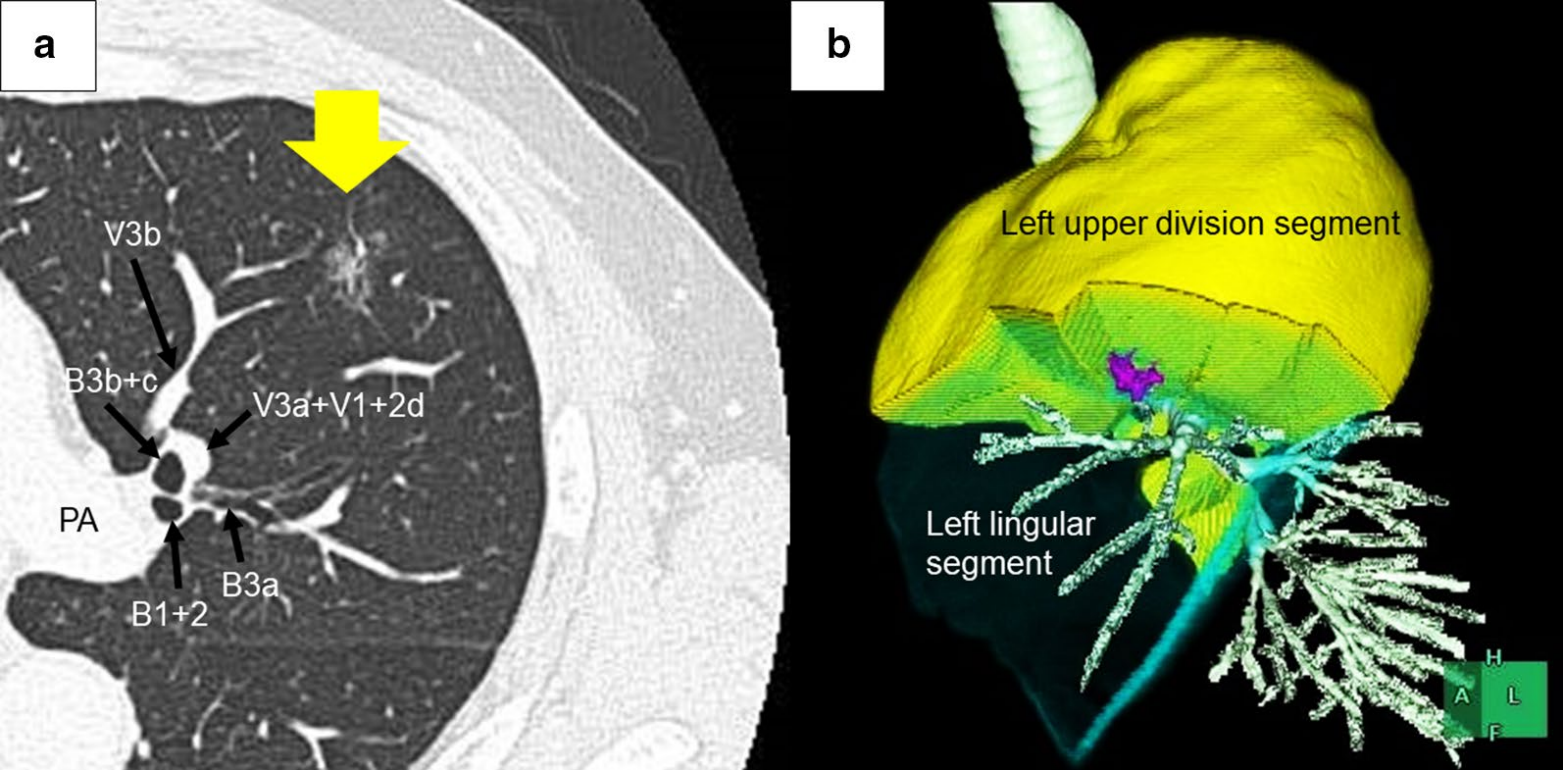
a Chest computed tomography reveals a partly solid, ground-glass nodule that is highly suspicious for malignancy. b Preoperative three-dimensional computed tomography reconstruction imaging reveals that the nodule (purple) is in the left lingular segment, but is close to the intersegmental plane between the left upper division segment and the left lingular segment
